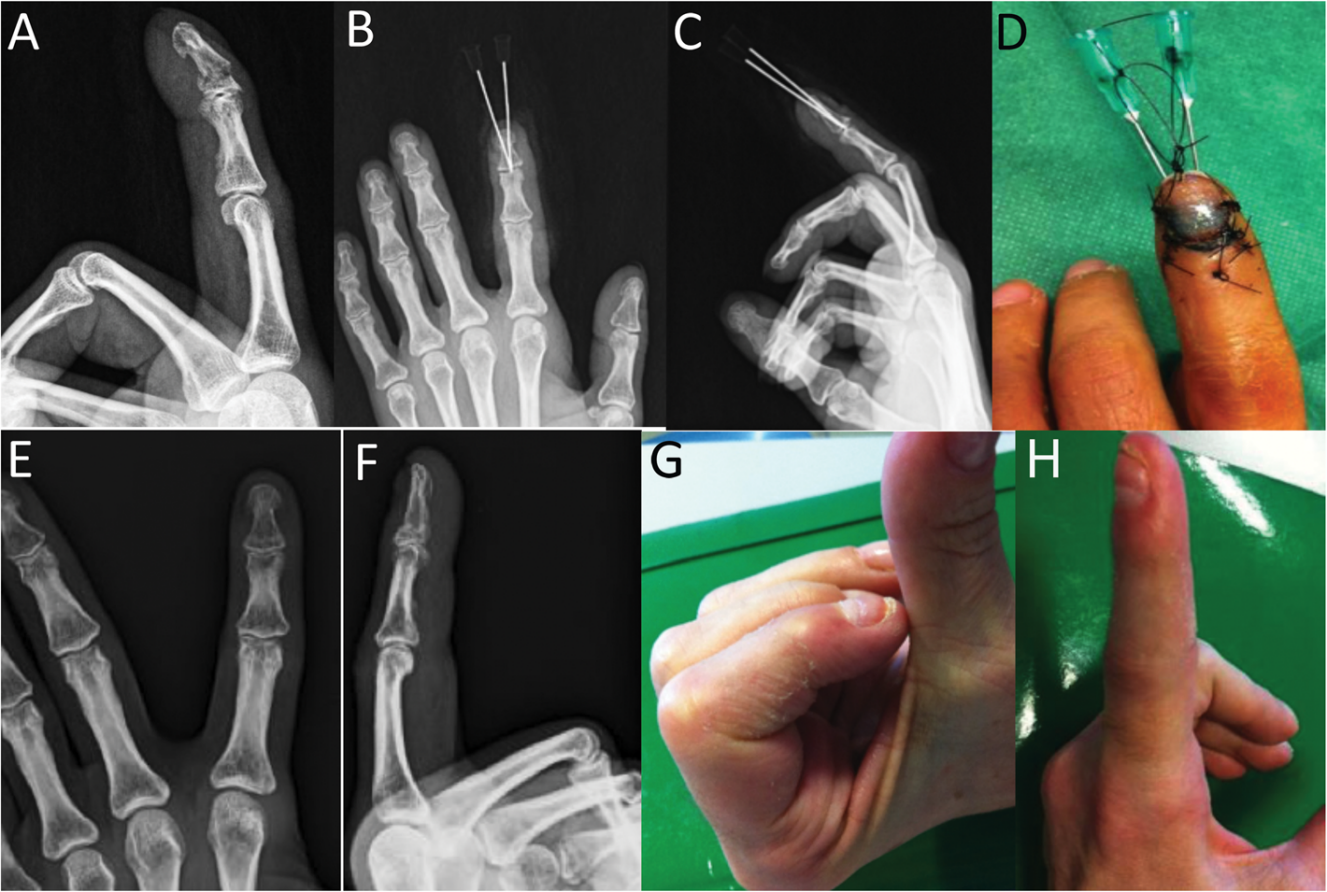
Female patient, 43 years old with crush injury of the tip of index finger and a shaft fracture (a) that was treated with 2 hand-crossed needles (b,c,d). Radiographic outcome after hardware removal 35 days postoperatively (e,f), and clinical outcome six months postoperatively (g,h)
